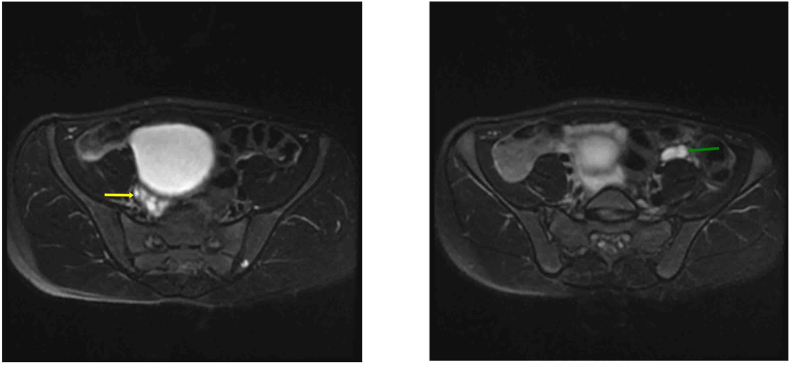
MRI pelvis, fat-suppressed T2WI axial sections in two different levels, show oval shape hyperintense structure on the right side "yellow arrow" and left side "green arrow" of the pelvis, that of right side contains small cystic foci which in favor of follicles inside an ovary.
